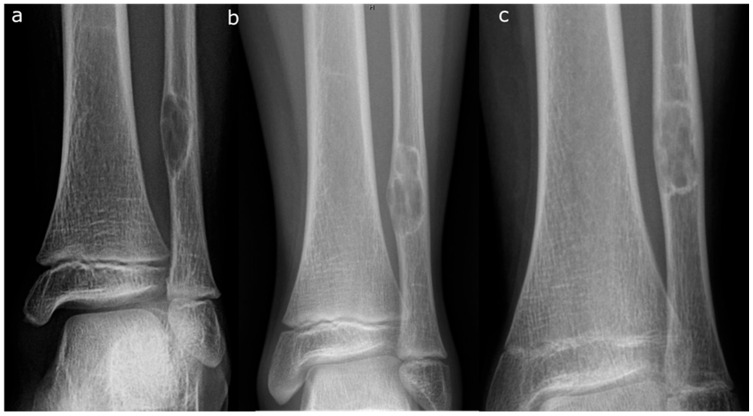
Evolution through Ritschl stages (a) An 8-year-old girl at the first presentation. AP radiograph showing a lytic lesion of the left distal fibula in stage A. (b) Two years later, the lesion was polycyclic in shape with sclerotic borders (stage B). (c) At the age of 12 years, there was evidence of ossification beginning at the diaphysis, representing a stage C lesion.
